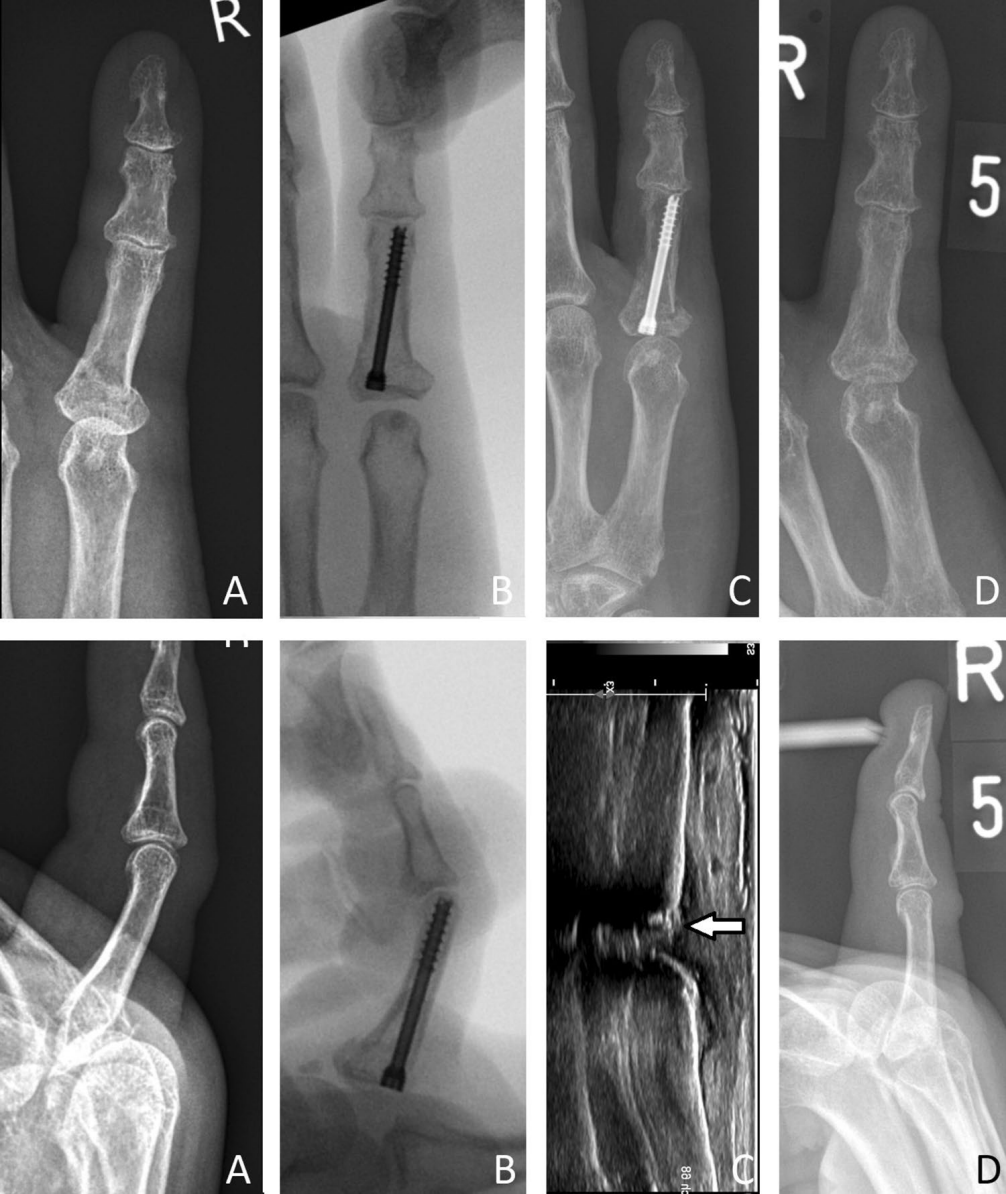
A First X-ray, B intraoperative radiographs, C 6 weeks postoperative. White arrow shows protruding screw in sonographic view, D after screw removal and healed fracture
